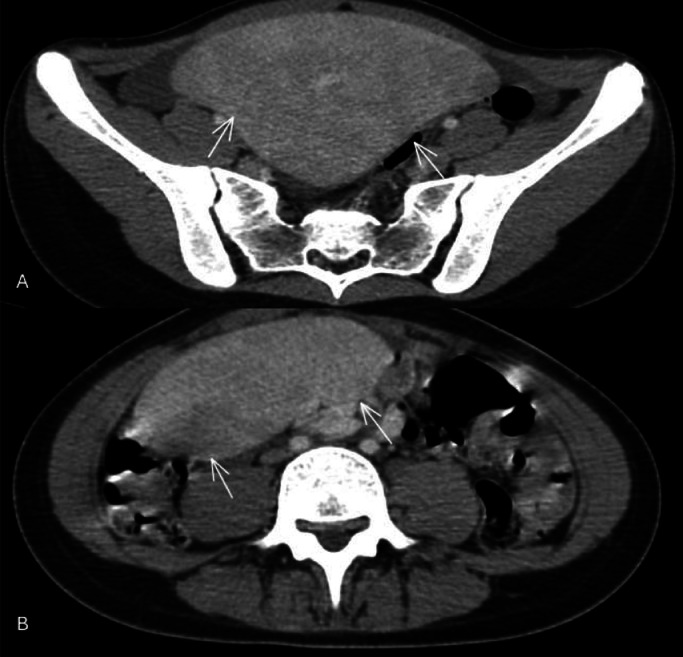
Axial (A) and (B) reformatted CT scan images of the abdomen post IV contrast administration, showing an ectopic spleen (white arrows) in the pelvic region.
